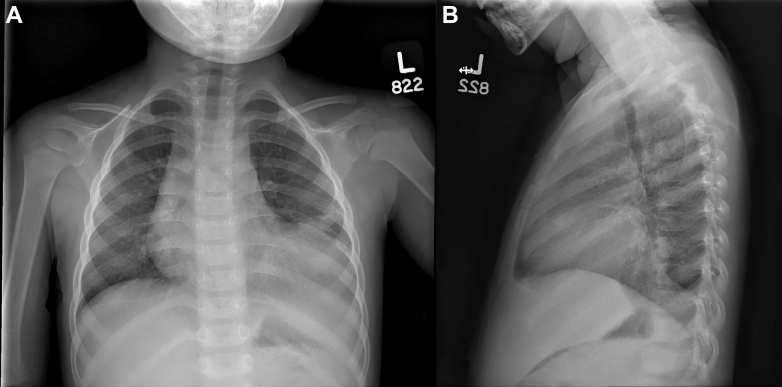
Chest X-ray Chest x-ray performed on day 1 of admission with coronal plane (A) and sagittal plane (B) showcasing a left lower lobe consolidation, addended upon review of the computed tomography scan to represent a pericardial cyst with associated streaky subsegmental atelectasis.
